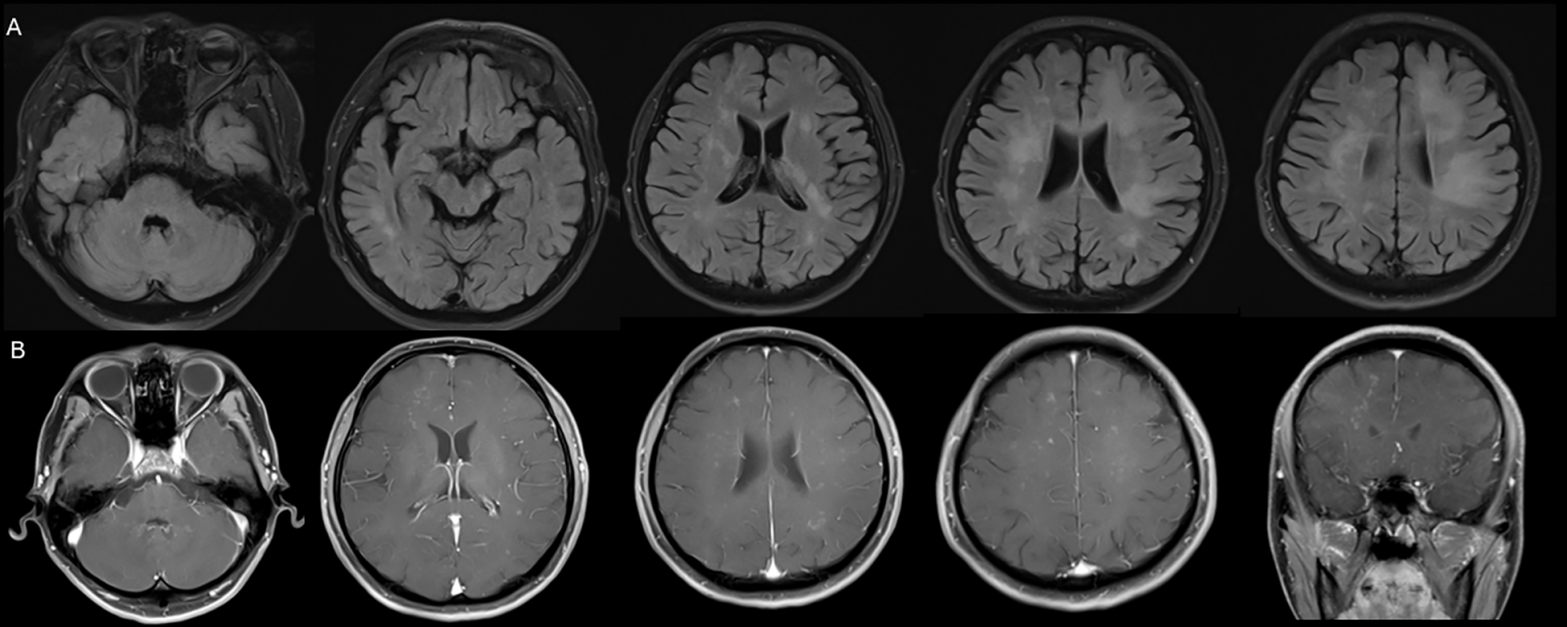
Neuroimaging studies of the patient. (A) Multiple patchy T2-FLAIR hyperintensities are noted within the bilateral cerebellar hemispheres, corpus callosum, pons, bilateral fronto-temporo-parieto-occipital lobes, thalamus, basal ganglia, corona radiata, and centrum semiovale. (B) On postcontrast sequences, multiple punctate enhancing foci are demonstrated within these regions.
